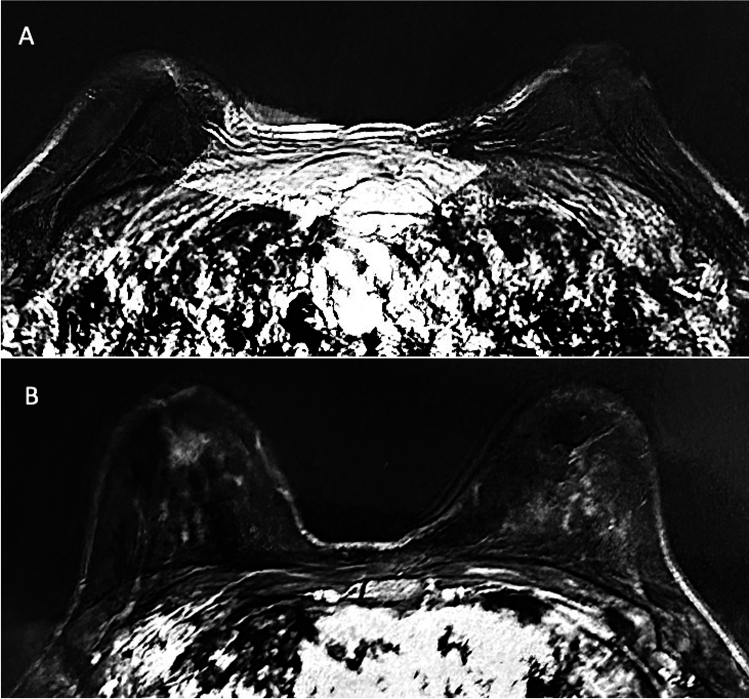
T2-weighted MRI scans of the SG patient showed in Fig. 1. A Pre-operative situation of the patient with a high degree of breast hypoplasia bilaterally. B Post-operative obtained 6 months later the second fat graft injection with significative volume improvement in the breasts bilaterally
